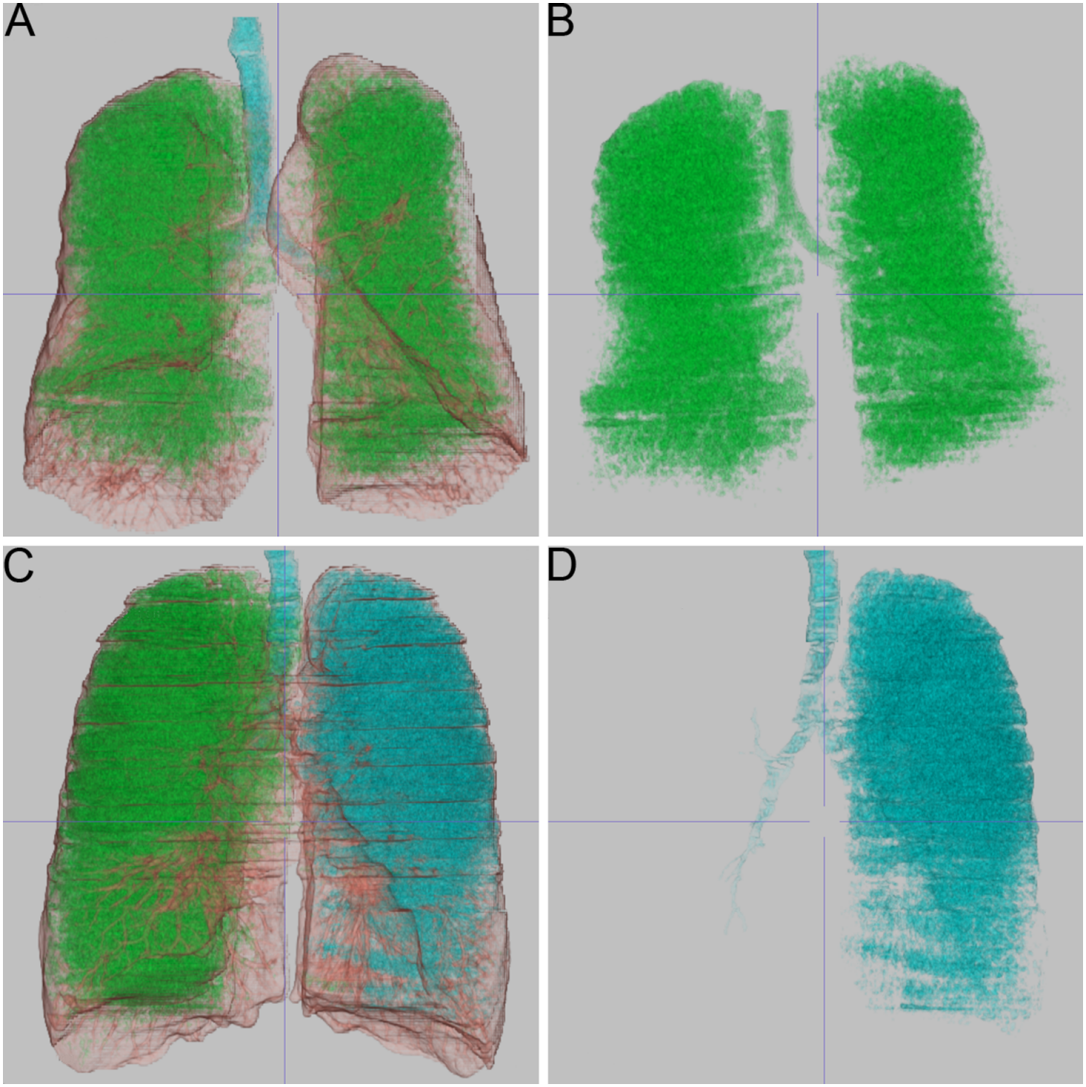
Emphysema visualization by density maps. Every software offered volume-rendering of segmented central airways (highlighted in blue), lung (brown) and emphysema (green). Leakage of the segmentation algorithms from airways into the parenchyma and vice versa are frequent sources of error in densitometry on computed tomography datasets, which results in faulty results or unexpected halt of the software tool. A, B 73–year-old patient, FEV1 = 43%, emphysema index calculated with 37% and 40%, not processed by the third software tool. A suggests correct segmentation of airways and emphysema, whereas isolated display of emphysema in B demonstrates that the software has assigned the airway tree actually also to the emphysema volume. C, D 52–year-old patient, FEV1 = 20%, emphysema index calculated with 41% and 45%, not processed by the third software tool. Visualization of the segmented airways and emphysema in C, and selective display of the segmented airways in D show that airway segmentation leaked into the left lung. Respiratory artifacts can be appreciated in C, which have obscured the airway wall of segmental airways on the left side.
